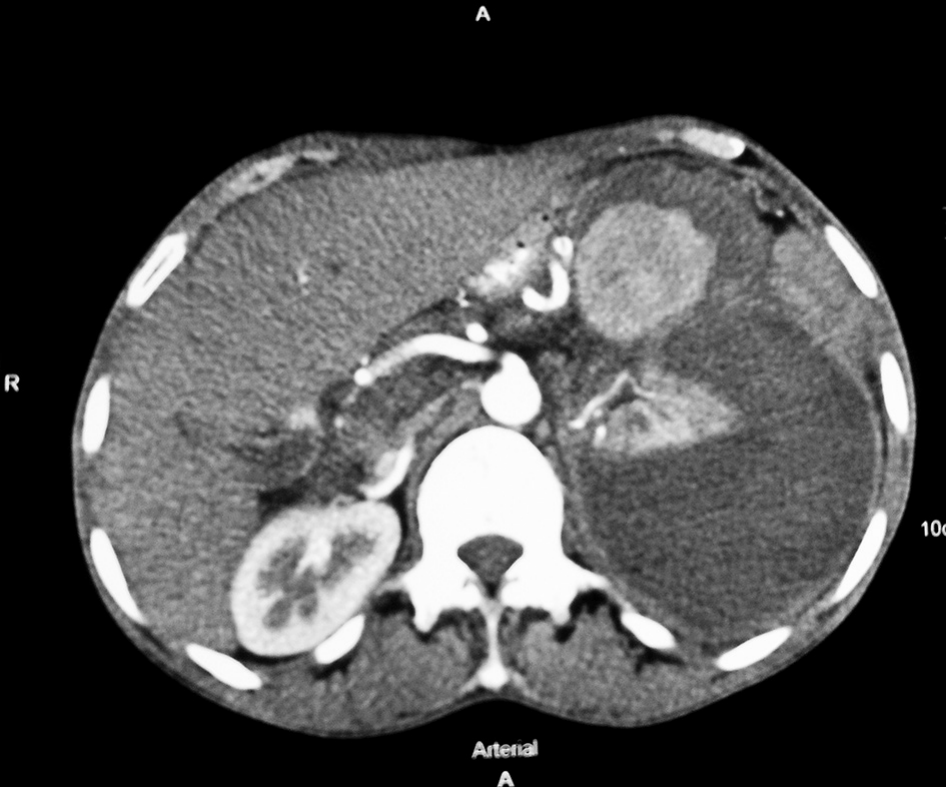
Contrast-Enhanced Computed Tomography of Abdomen Showing Large Splenic Aneurysm With Large Perinephric Hematoma Compressing the Kidney
